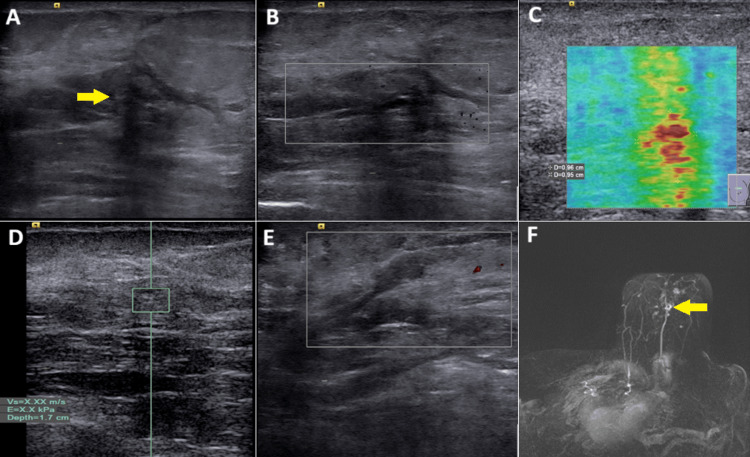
One year post-ablation. (A) Greyscale ultrasound showing an area of architectural distortion at the ablation site with no evidence of a tumor residue (arrow). (B) Color Doppler interrogation showing no vascularity. (C) Qualitative ultrasound elastography demonstrating an area of high stiffness (red) over the architectural distortion. (D) Quantitative ultrasound elastography showing very high stiffness above the upper limit (≥6.5 m/s), recorded as X.XX m/s. (E) Color Doppler interrogation showing no vascularity and architectural distortion for the metastatic appearing left axillary lymph node. (F) MRI showing focal fat necrosis in the region of the ablated region (arrow) and the absence of the left axillary lymph node metastasis
